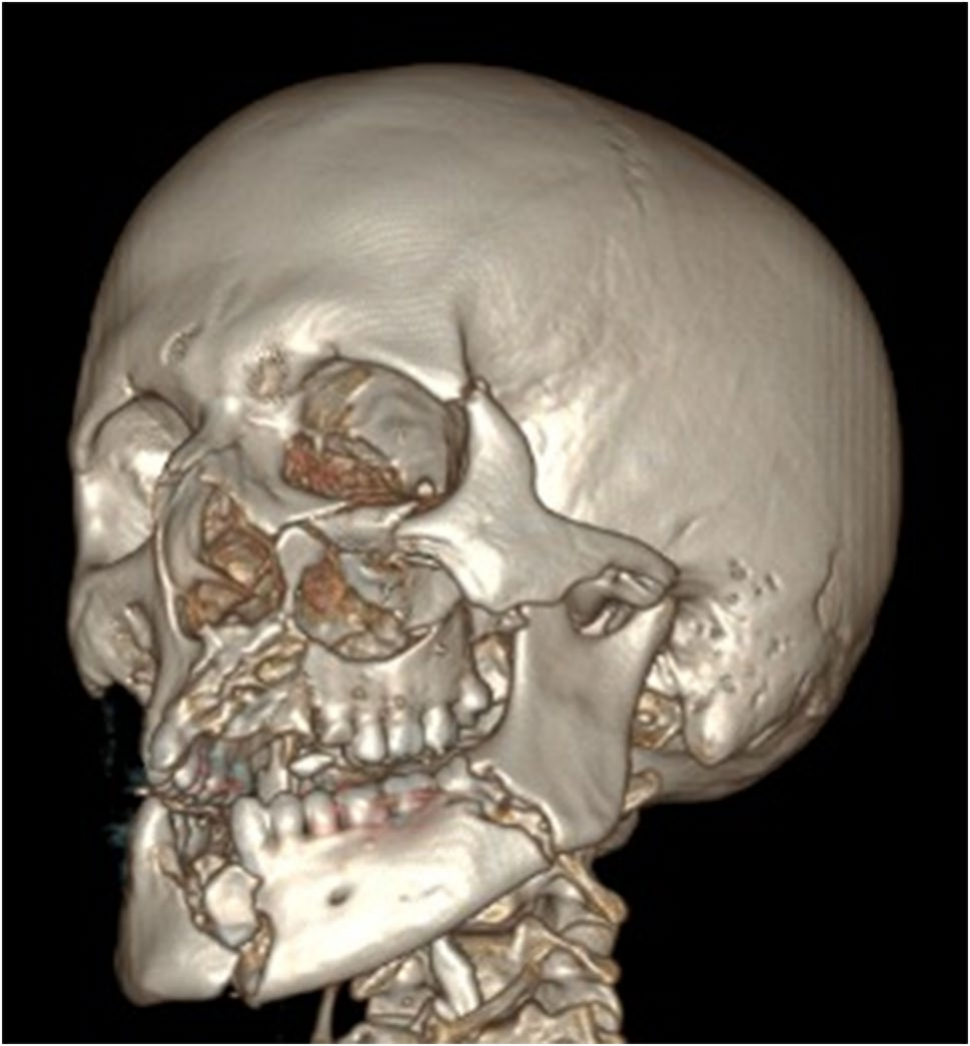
CT imaging–based 3D reconstruction of the mid face and skull
